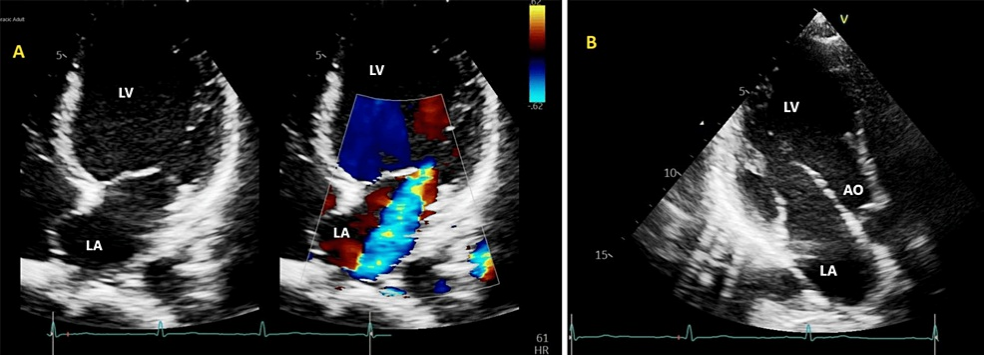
Follow-up transthoracic echocardiogram images (A) Mitral regurgitation in the color Doppler view; (B) View of the left atrium (LA), left ventricle (LV), and aorta (AO).
